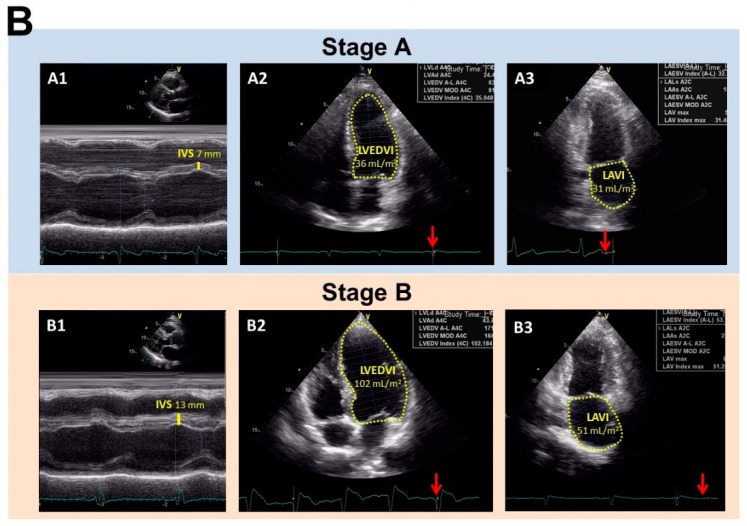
Representative echocardiographic images and the NT-proBNP levels of subjects in Stage A and B heart failure (HF). (A) The NT-proBNP levels of the subjects in Stage A and B HF. * indicated *p* < 0.05 by independent *t*-test. (B) Upper panel: a 68-year-old male subject with Stage A HF (body surface area (BSA) of 1.8 m^2^). His echocardiographic parameters, including interventricular septum thickness (IVS; A1, 7 mm, normal range 6–10 mm), left ventricle end diastolic volume index (LVEDVI; A2, 36 mL/m^2^; normal range < 95 mL/m^2^), and left atrium volume index (LAVI; A3, 31 ml/m^2^; normal range < 34 mL/m^2^) were within normal ranges. Bottom panel: a 70-year-old male subject with asymptomatic Stage B HF (BSA 1.9 m^2^). The echocardiographic examination results demonstrated myocardial remodeling, including thickened IVS (B1, 13 mm) and enlarged LVEDI (B2, 102 mL/m^2^) and LAVI (B3, 51 mL/m^2^). The red arrow indicates that the echocardiographic pictures were taken at the end-diastolic phase in A2 and B2, and at the end-systolic phase in A3 and B3.
